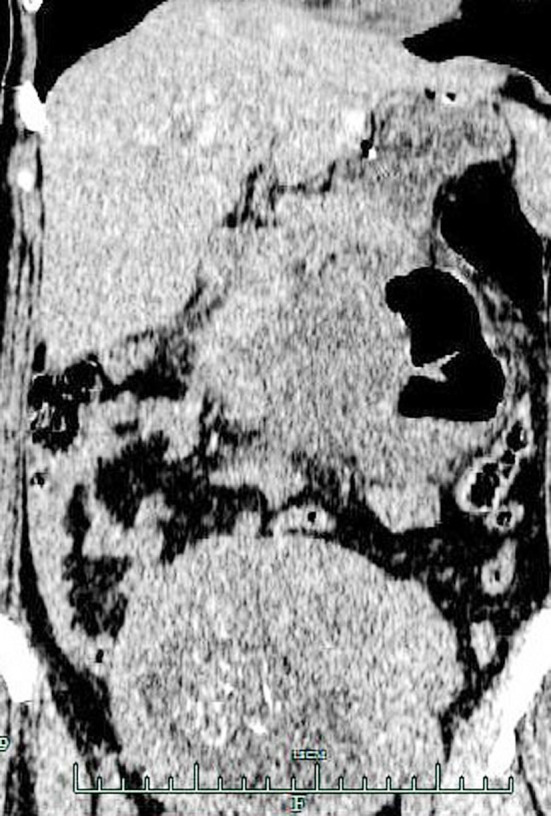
Coronal CT image of the abdomen shows dilated small bowel loops and air-fluid level with herniating mass. Gestational uterus is also seen.
